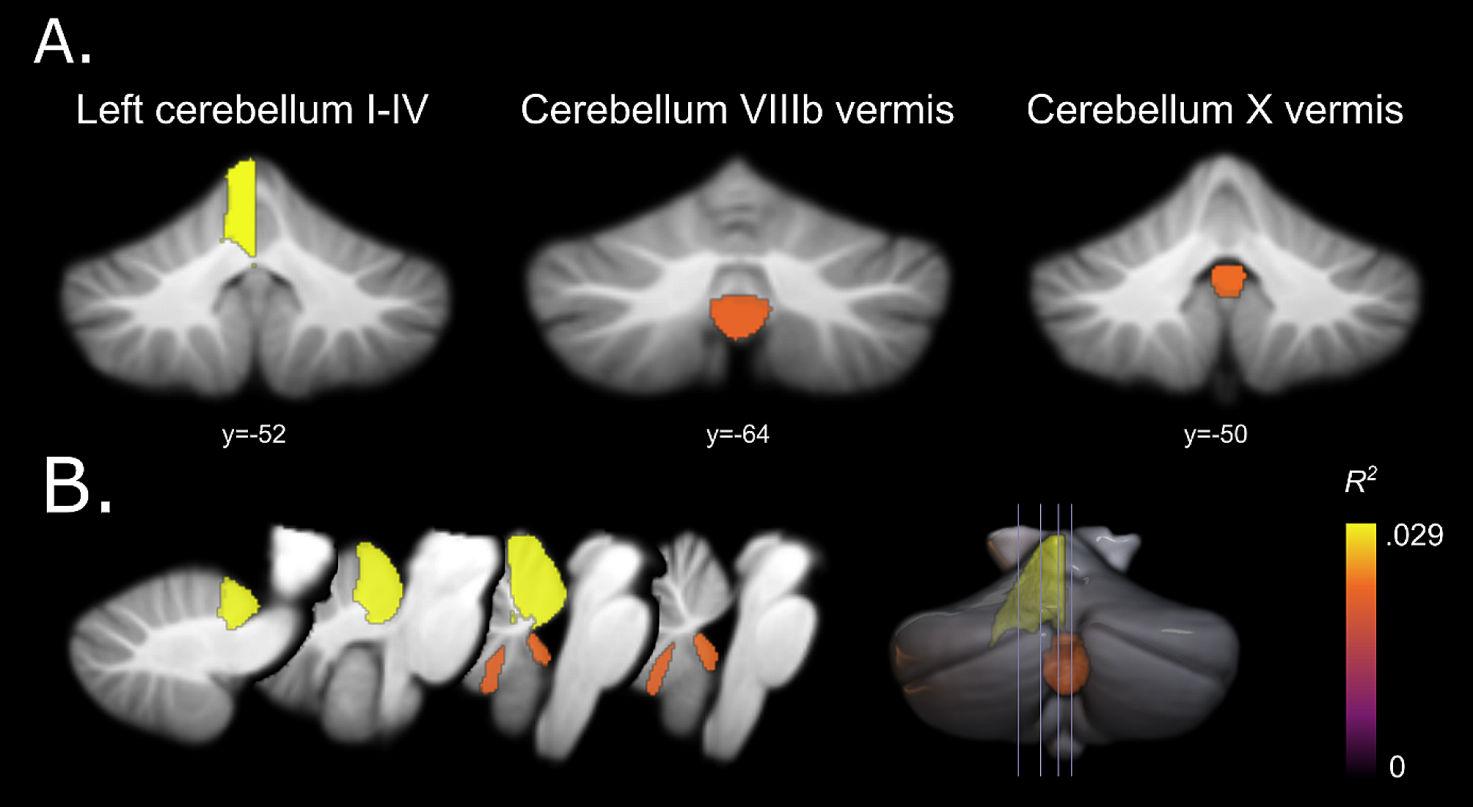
Anatomical localization of polygenic risk effects for ASD on the secondary MRI phenotypes, *Sub-regional Volumes*. (A) Coronal cerebellum sections, (B) sagittal sections and render view (right) depicting the significant association between the cerebellar sub-regional volumes and ASD PRS. The color bar indicates the highest variance (*R*^*2*^) explained by the ASD PRS for the secondary MRI phenotypes with significant associations
